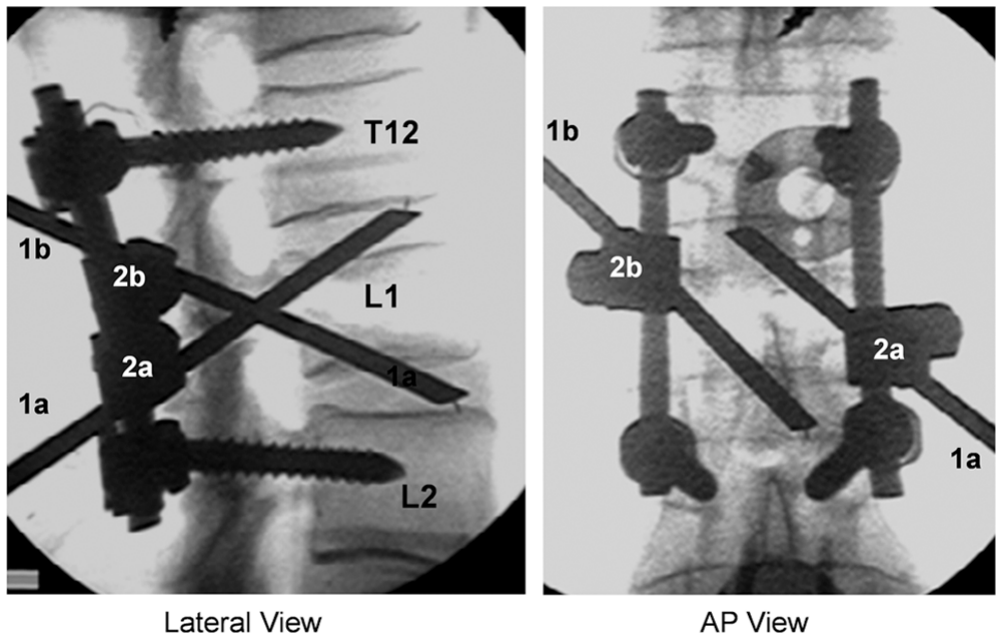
Radiograph of SSPI+TEPPS (Moss Miami System Combined With Trans-Endplate Pedicle Pillar System). Radiograph of fractured spine in L1 reveals SSPI+TEPPS instrumentation. Left, Lateral view shows the upper endplate pillar (1a) with its connector (2a) and the lower endplate pillar (1b) with its connector (2b). Right, Anteroposterior view shows the upper endplate pillar (1a) with its connector (2a) and the lower endplate pillar (1b) with its connector (2b).
